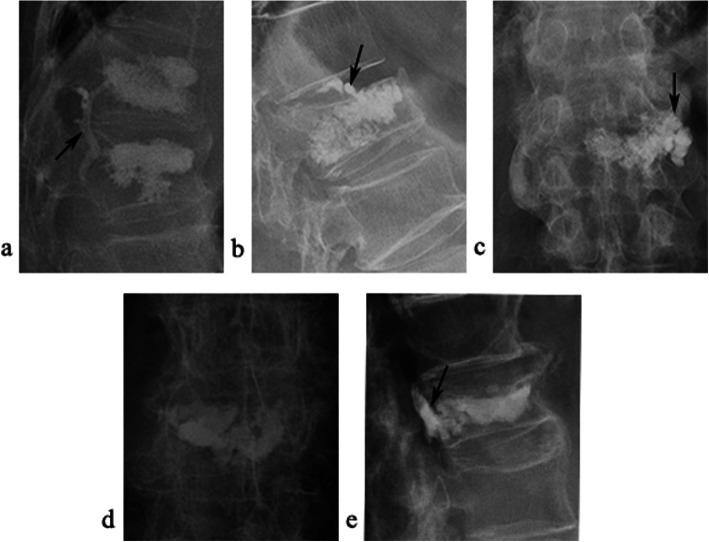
Imaging manifestation of different types vertebral cement leakage. Postoperative X-ray a lateral position X-ray reveals the cement vein leakage (arrow) b lateral position X-ray reveals the intradiscal cement leakage (arrow) c anterior-posterior (AP) position X-ray reveals the cement paravertebral leakage (arrow) d and e AP and lateral position X-ray revealed the cement spinal canal leakage (arrow)
